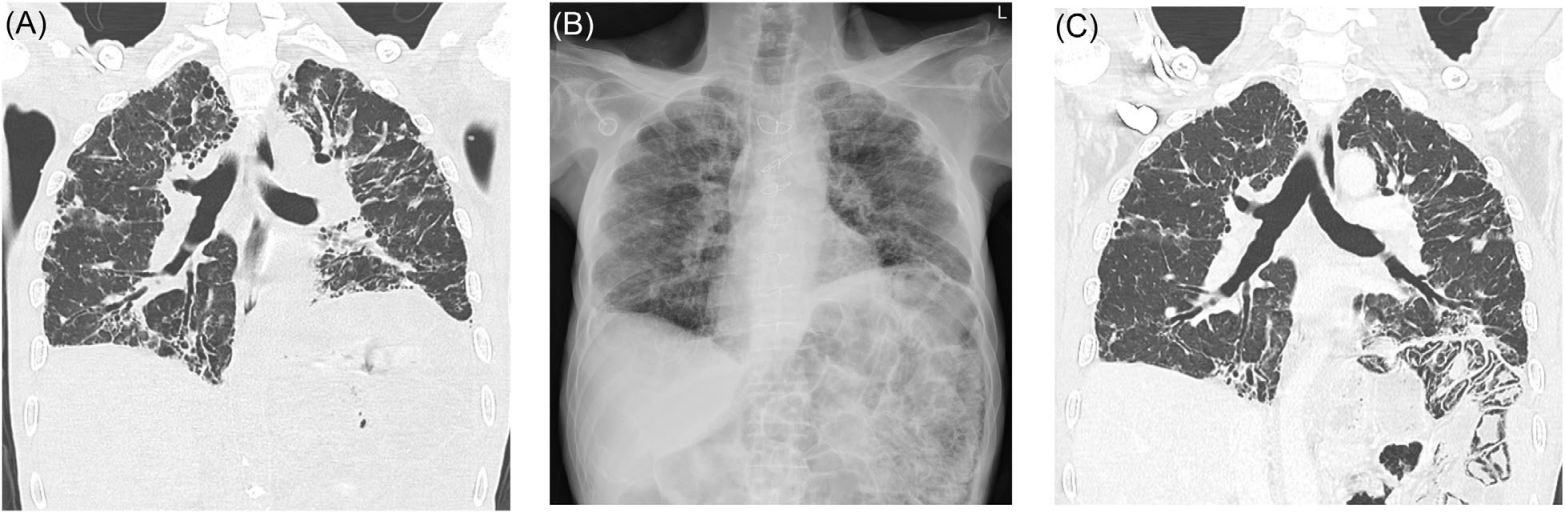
(A) A representative coronal‐view thoracic computed tomography (CT) image of the patient showing bilateral multi‐lobar bronchiectasis. (B) The follow‐up chest radiograph and (C) coronal‐view CT image incidentally revealed pneumatosis intestinalis, but not any evidence of pneumothorax or pneumomediastinum.
